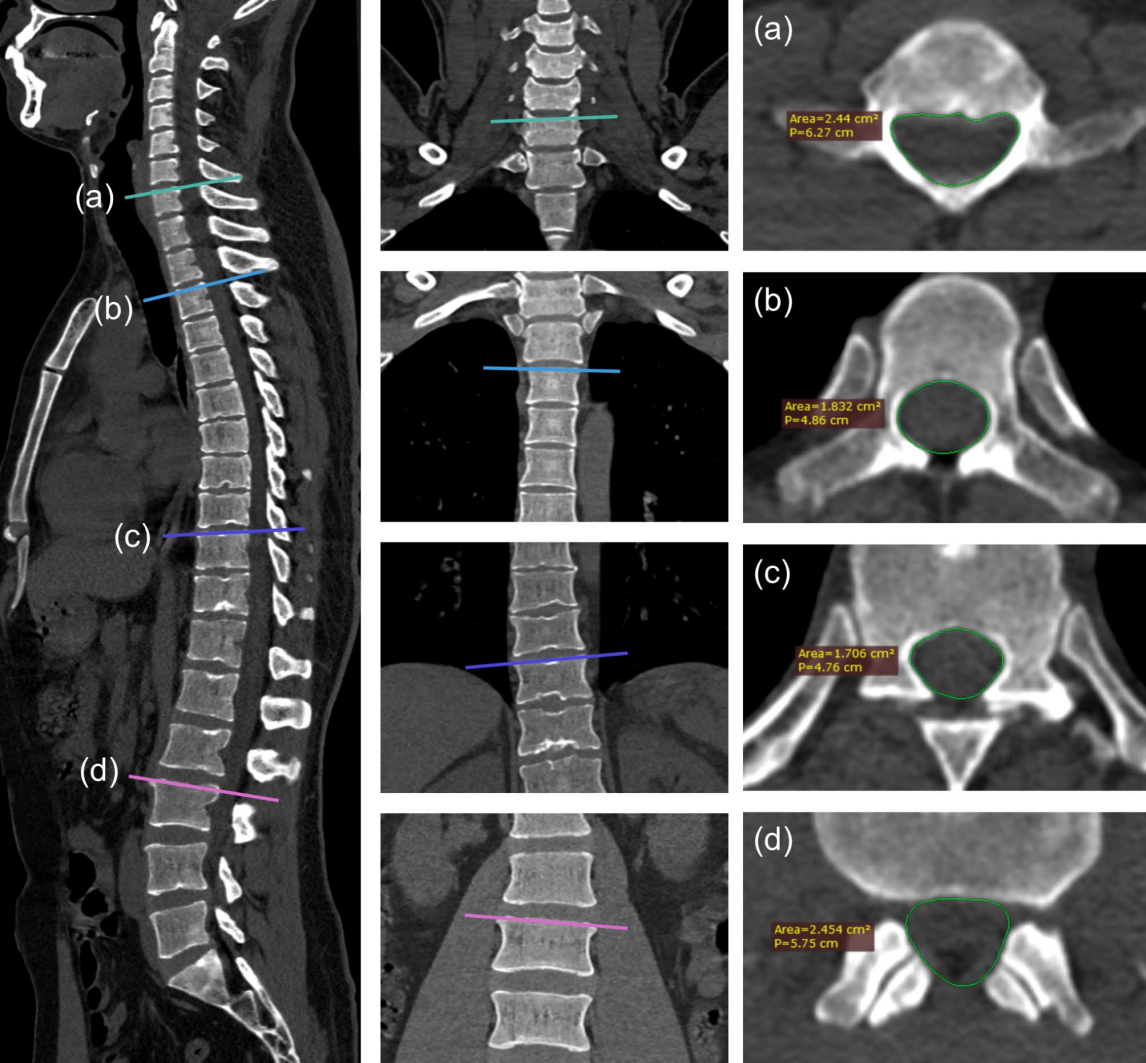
Measurement of spinal canal cross-sectional area at different levels. The left and middle panels show sagittal and coronal views, with colored lines indicating the measurement planes (a-d). The right panel presents the corresponding axial CT slices, where the spinal canal cross-sectional area was manually delineated (green outline). (a) C6-7 level. (b) T2-3 level. (c) T9-10 level. (d) L2-3 level
